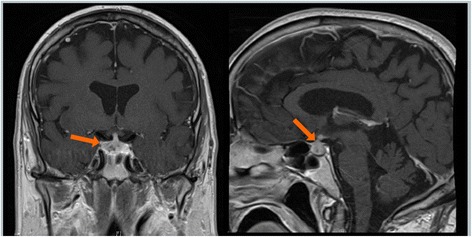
MRI brain two months prior to onset of visual complaints, demonstrating enlargement and enhancement (arrows) of the pituitary gland consistent with hypophysitis
